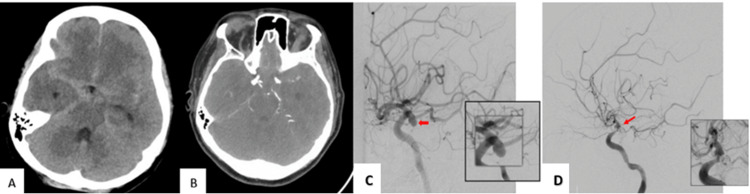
(A) Non-contrast computed tomography scan of the brain showed diffuse subarachnoid haemorrhage; (B) CT angiogram and (C) catheter angiogram showed the right posterior communicating artery aneurysm; (D) Post-embolization angiogram showed protection of the dome of the aneurysm
